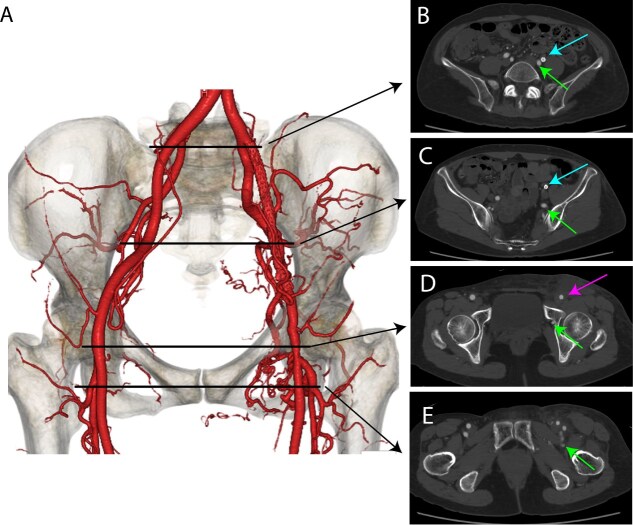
Postoperative contrast-enhanced CT images. (A) VR image. (B) Bifurcation level of the external iliac artery (blue arrow) and the internal iliac artery. The sciatic artery is marked with a green arrow. Improved blood flow is observed in the left external iliac artery. (C) Pelvic level. The external iliac artery (blue arrow) and the sciatic artery (green arrow) are shown. Improved blood flow is observed in the left external iliac artery. (D) Femoral head level. The left common femoral artery (pink arrow) and the sciatic artery (green arrow) are displayed. The lumen of the left common femoral artery appears widened. (E) Postbifurcation of the superficial femoral artery and deep femoral artery. The sciatic artery (green arrow) is visible.
